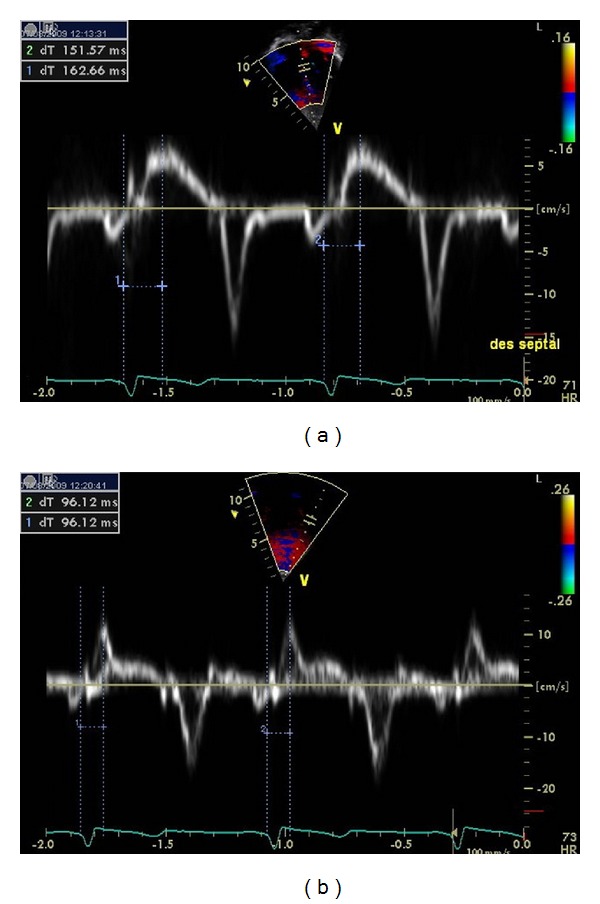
Determination of the delay between time-to-peak systolic velocity at basal septal (a) and basal lateral (b) segments using tissue Doppler imaging. A significant delay is demonstrated in this patient (no. 10).
